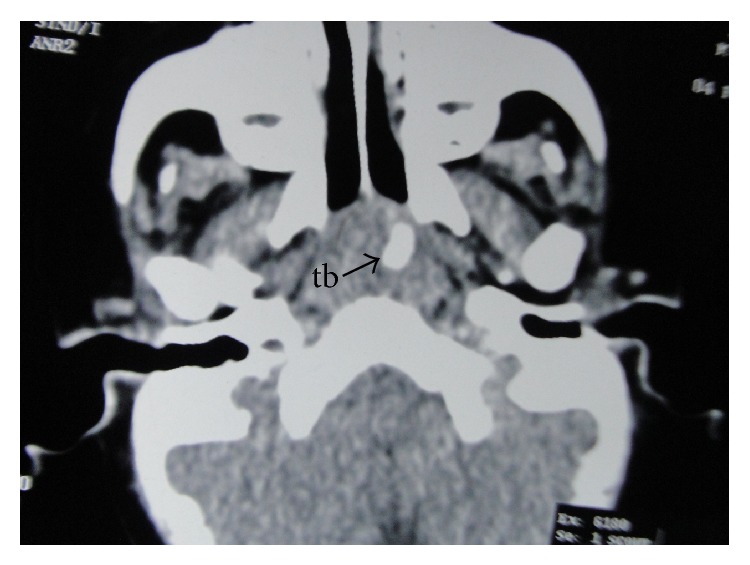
CT scan passing through the external auditory meatus, showing the handle of the toothbrush (tb) in the retropharyngeal space of the nasopharynx.
